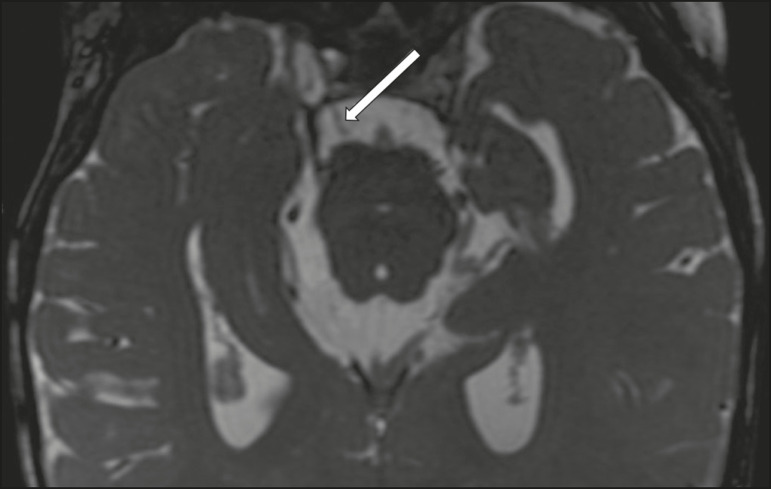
Absence and hypoplasia of the abducens nerve. A one-month-old male with congenital lateral rectus muscle palsy and globe retraction and presenting upshoot and downshoot with certain eye movements, therefore being diagnosed with Duane syndrome. Axial 3D-SSFP MRI sequence showing the right abducens nerve (arrow), whereas the left one is not visible.
